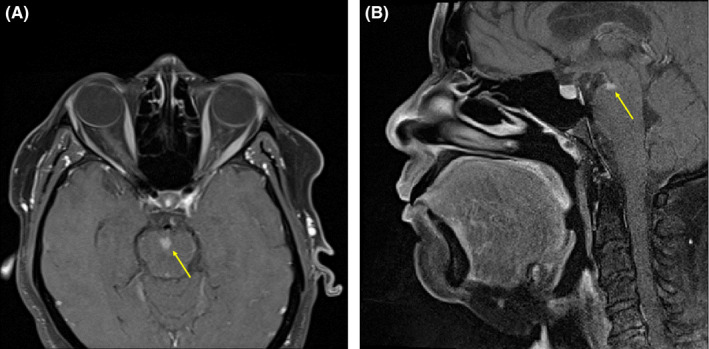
Axial (Panel A) and sagittal (Panel B) postgadolinium T1‐weighted brain MRI revealed a new enhancing lesion in the right superior pons (arrows), ipsilateral to the patient's perioral myokymia
